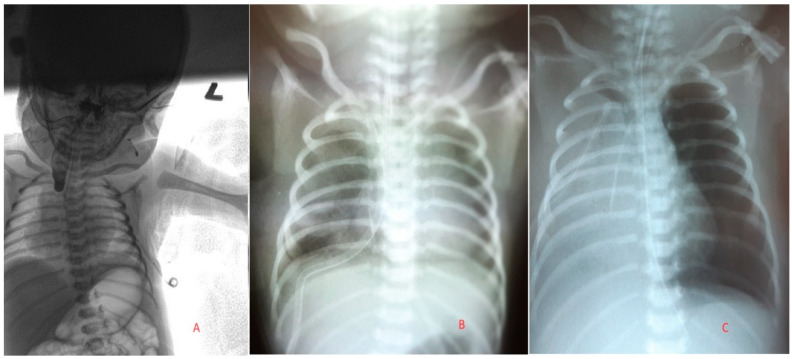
(A)—X-ray-showing the upper end of the esophagus at the level of T4-5, dilated intestinal loops filled with air. (B)—X-ray image taken 2 h post-operation. (C)—X-ray taken 6 days after surgery and after re-drainage of the chest.
